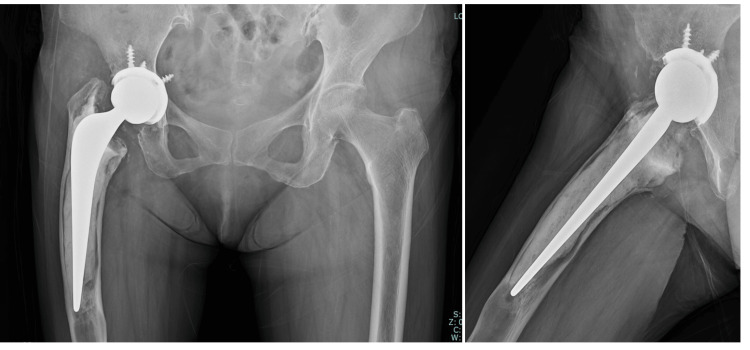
Follow-up radiograph at three years postoperatively Radiograph taken at the three-year follow-up showing stable fixation of the cemented femoral stem without signs of loosening, periprosthetic fracture, or osteolysis. The patient remained asymptomatic with independent ambulation.
